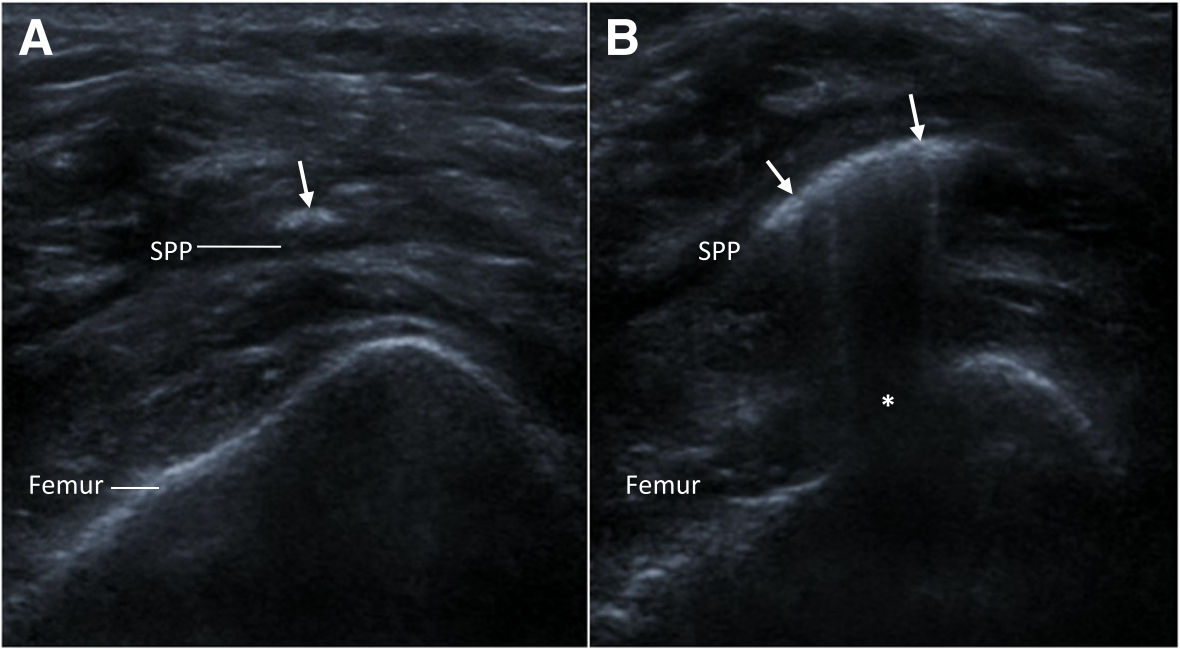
A transverse ultrasound section of the mid supra-patellar pouch area of the right knee during the very early (a) and later (b) phase of injection, showing a positive air arthrosonogram. There is an emerging area of intra-articular air bubbles (arrows), initially faintly (a) and then more clearly visible (b), with posterior acoustic shadowing (*). Varying transducer pressure demonstrated the echogenic layer to be displaceable and display buoyancy, consistent with intra-articular placement of the injection. SPP denotes suprapatellar pouch
